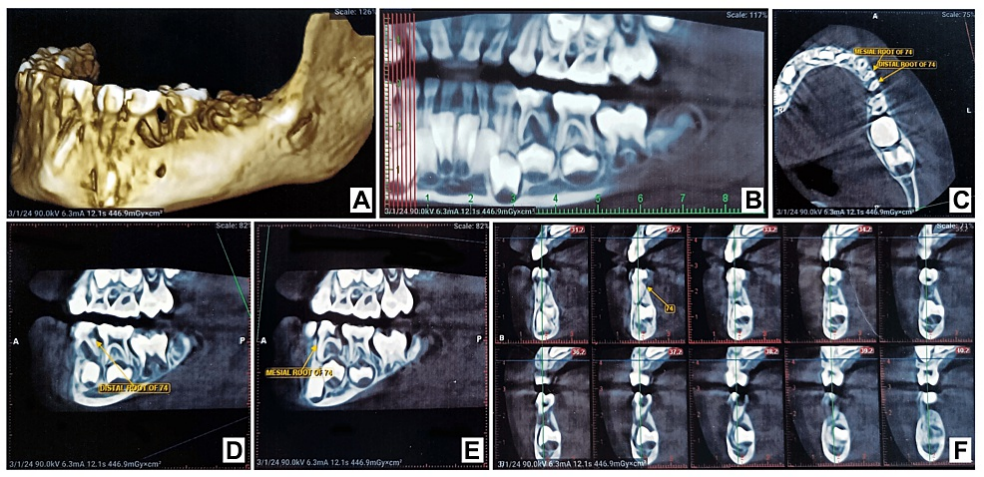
CBCT image showing disto-proximal caries and interradicular bone loss with 74 Sub-images A and B showing disto-proximal caries and interradicular bone loss with 74. Sub-images C, D, E, and F show various aspects of the mesial and distal roots with 74.
